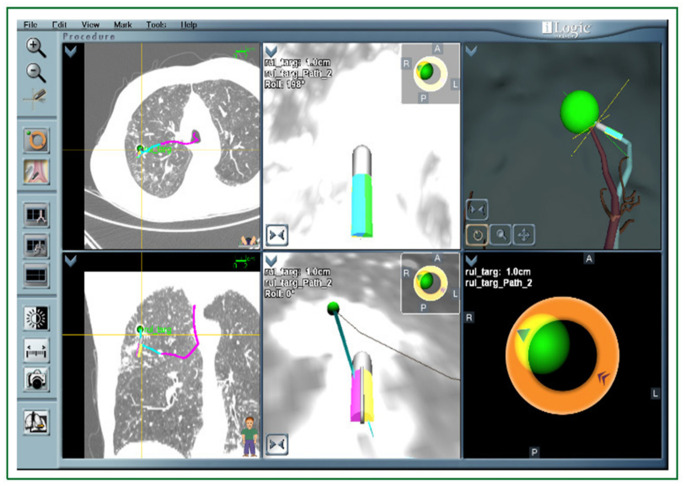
Electromagnetic navigation bronchoscopy (superDimension^®^) with extended working channel and locatable guide tracked against a pre-acquired CT roadmap [reused with permission from AME Publishing].
